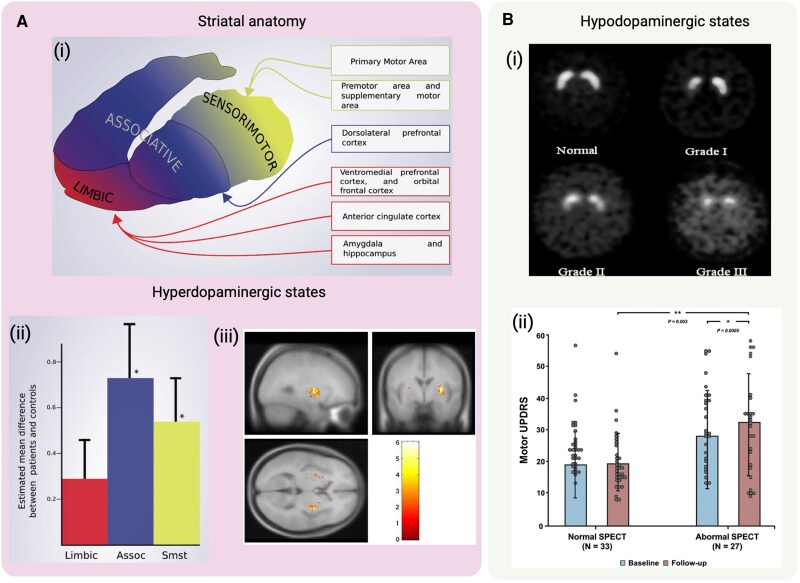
Dopamine and the striatum in schizophrenia. [A(i)] Functional divisions of the striatum with corresponding inputs. (ii) Cumulative effect sizes for elevations in dopamine synthesis or release capacity across subdivisions of the striatum, from a meta-analysis of seven PET ligand studies comparing patients with schizophrenia and controls.^106^ Panels A(i and ii) reproduced from McCutcheon *et al*.^106^ under the terms of Creative Commons Attribution License (CC BY 4.0). (iii) ^18^F-DOPA PET images demonstrate increased dopamine synthesis capacity within the sensorimotor striatum (Smst) in patients with prodromal features prior to psychosis onset compared with healthy controls.^102^ Increased dopamine synthesis in the sensorimotor striatum was most strongly correlated with development of psychosis in this cohort (reproduced with permission from Howes *et al*.^102^) [B(i)] Dopamine transporter (DaT) SPECT images from medicated patients with schizophrenia and parkinsonism and various grades of nigrostriatal denervation (Normal, Grades I–III). Reproduced from Tinazzi *et al*.^114^ with permission from Elsevier. (ii) Patients with schizophrenia and parkinsonism demonstrating abnormal DaT imaging exhibit progressive decline in motor symptoms of parkinsonism whilst those with normal DaT do not show progression. Reproduced from Tinazzi *et al*.^115^ with permission from Elsevier. Created in BioRender. Joseph, A. (2026) https://BioRender.com/cuogt7h. SPECT = single-photon emission computed tomography; UPDRS = Unified Parkinson's Disease Rating Scale.
